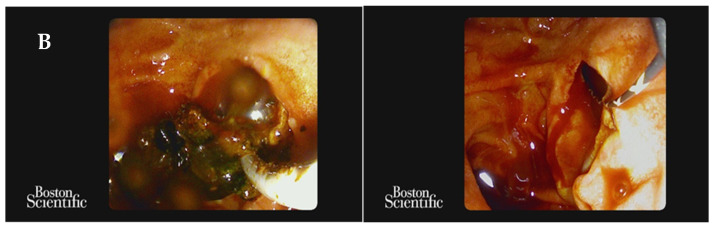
(A,B): X-ray image (A) and endoscopic view (B) of ERCP procedures and stones removal with Exalt D Single-Use Duodenoscope in a fragile patient prone to develop infection.
